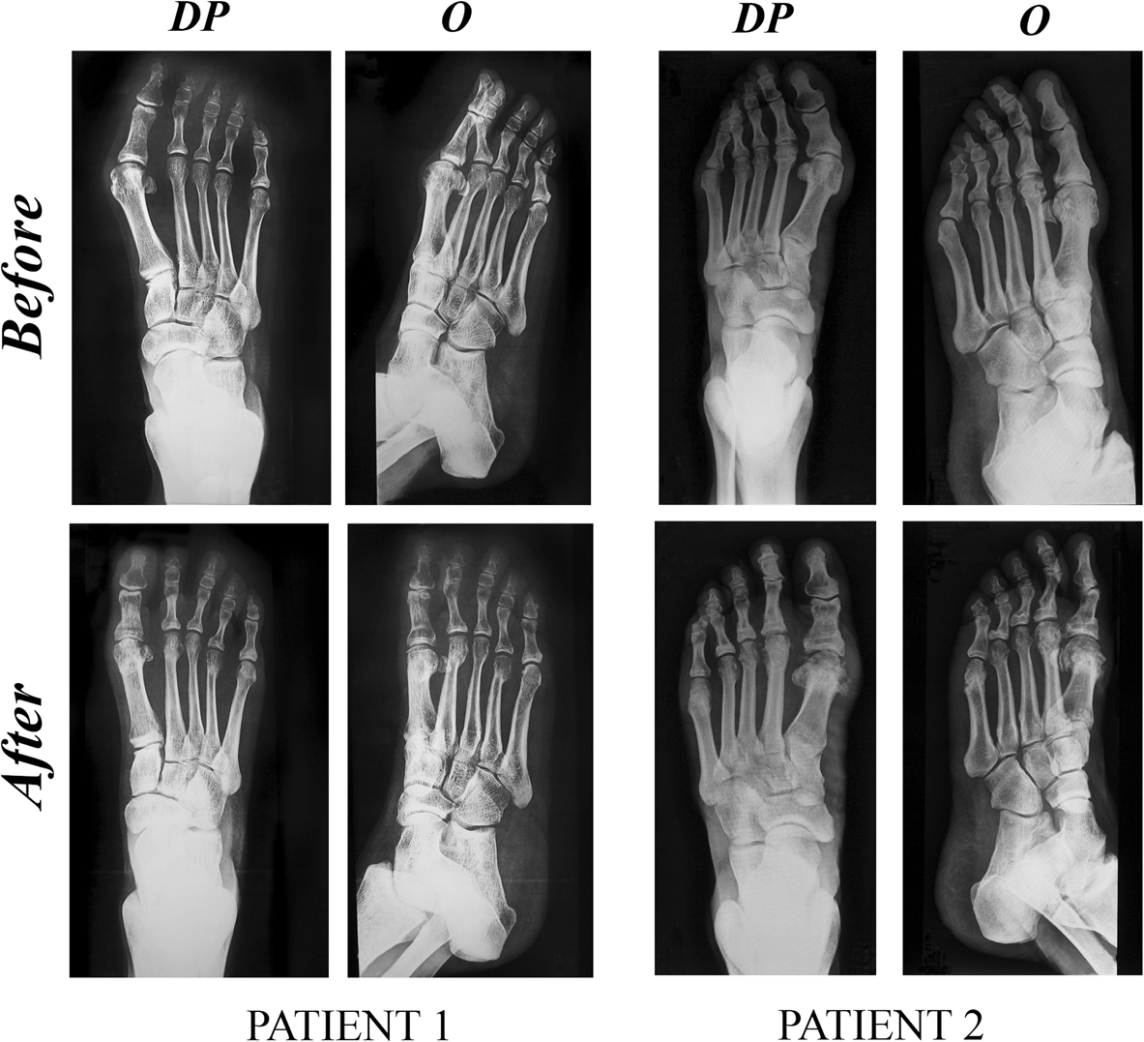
Examples of X-ray plates taken before and after surgical procedure from two patients (PATIENT 1 and PATIENT 2). DP dorsoplantar, O oblique
